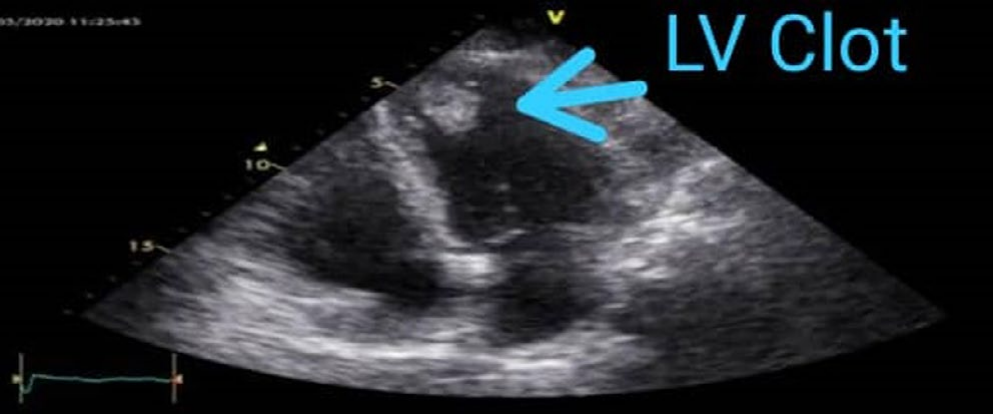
Case 11. Echocardiography shows a hypermobile clot in the LV (1.5 × 1.5 cm)
